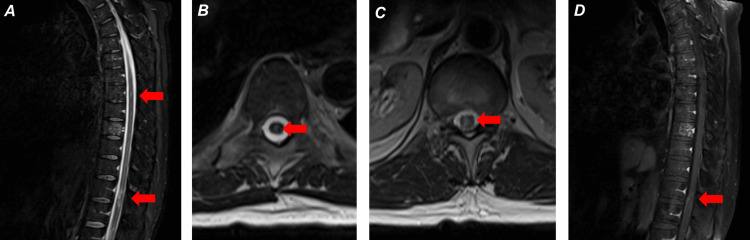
First post-fingolimod MS exacerbation: (A) (sagittal thoracic spine) T2/STIR signal hyperintense lesions at T7 (top red arrow) and T12-L1 (bottom red arrow), (B) (axial thoracic spine) T2/STIR signal hyperintense lesion at T7 (red arrow), (C) (axial thoracic spine) T2/STIR signal hyperintense lesion at T12-L1 (red arrow), and (D) (sagittal thoracic spine) T1 contrast enhancement at the T12-L1 lesion (red arrow). No enhancement at the T7 lesion (not pictured). MS: multiple sclerosis; T2/STIR: T2-weighted short-tau inversion recovery
